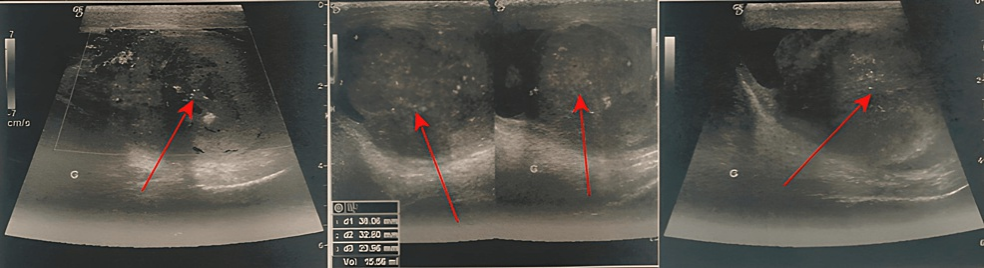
Ultrasound images showing a left testicular mass
